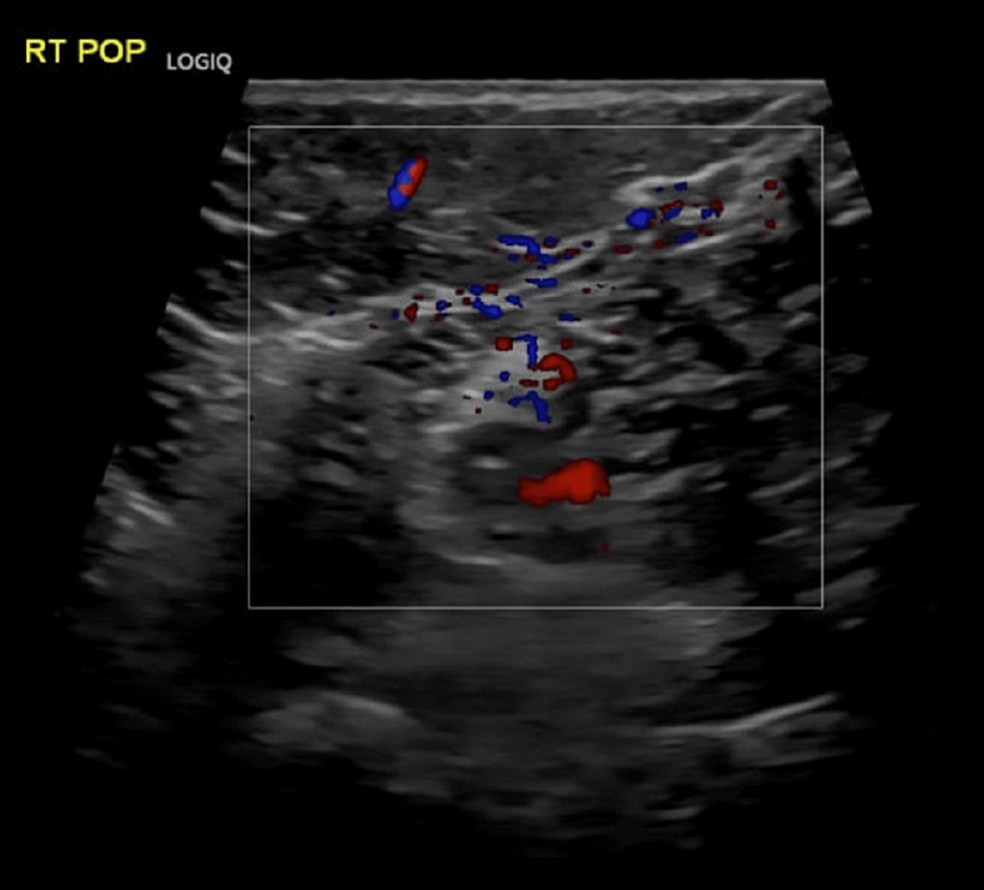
Doppler Ultrasound of the Right Popliteal Vein Ultrasound shows acute and subacute deep vein thrombosis in the right popliteal vein.
